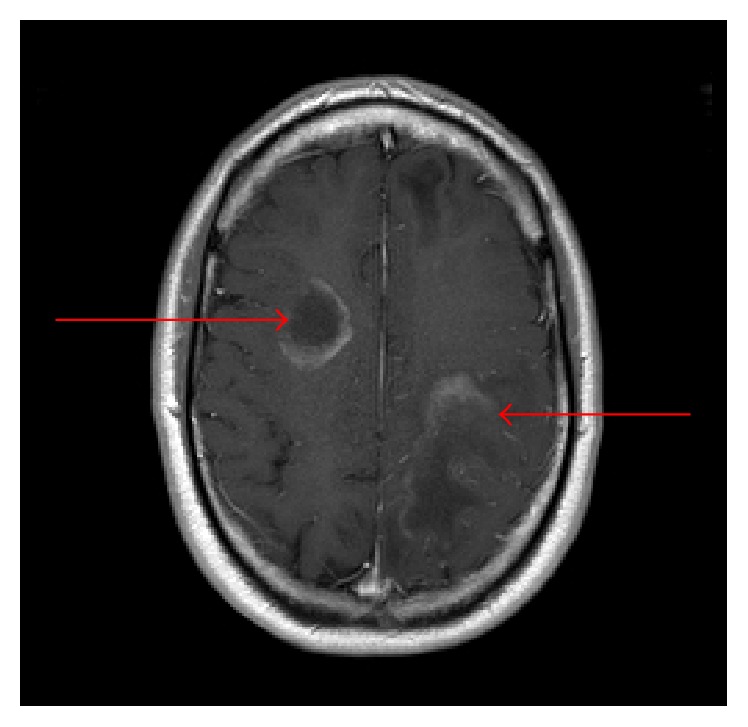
Brain MRI with bilateral peripherally enhancing white matter lesions representing signs of active demyelination.
